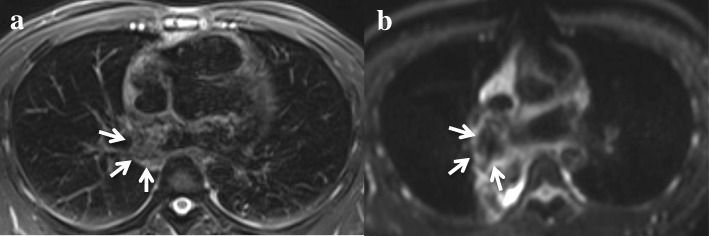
Local recurrence on magnetic resonance imaging (MRI). a At 7 months after surgery, before proton beam radiotherapy, recurrent tumor shadow was detected (arrows). b After proton beam radiotherapy, the tumor shadow was hardly detected (arrows)
